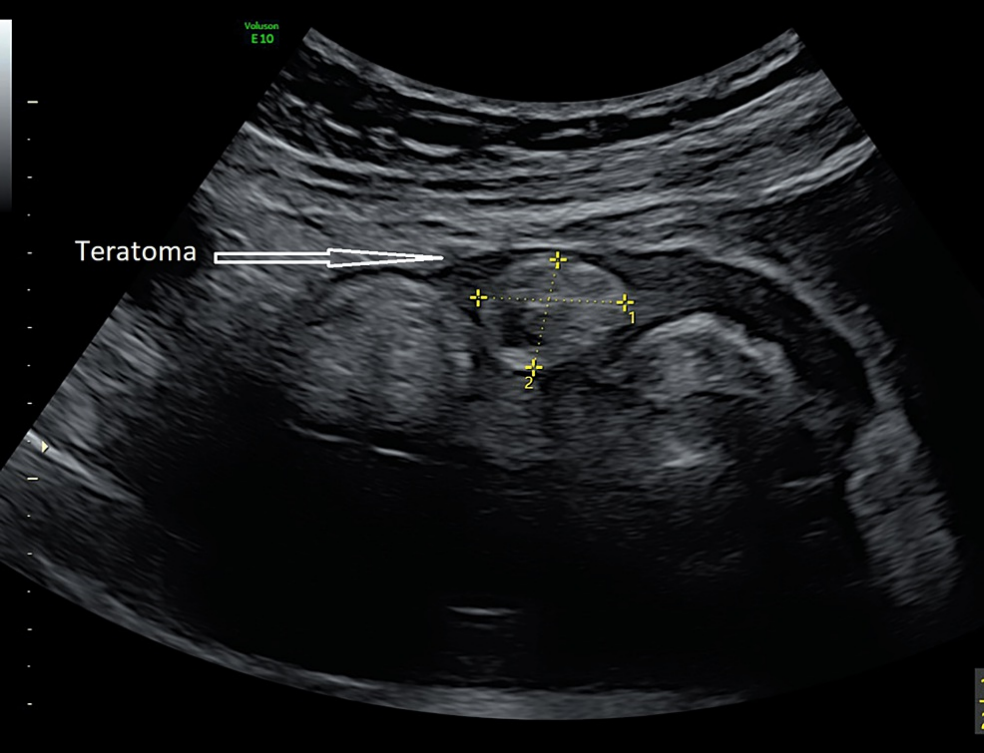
USS image of the pelvis showing a teratoma with fatty tissue USS: ultrasound scan
